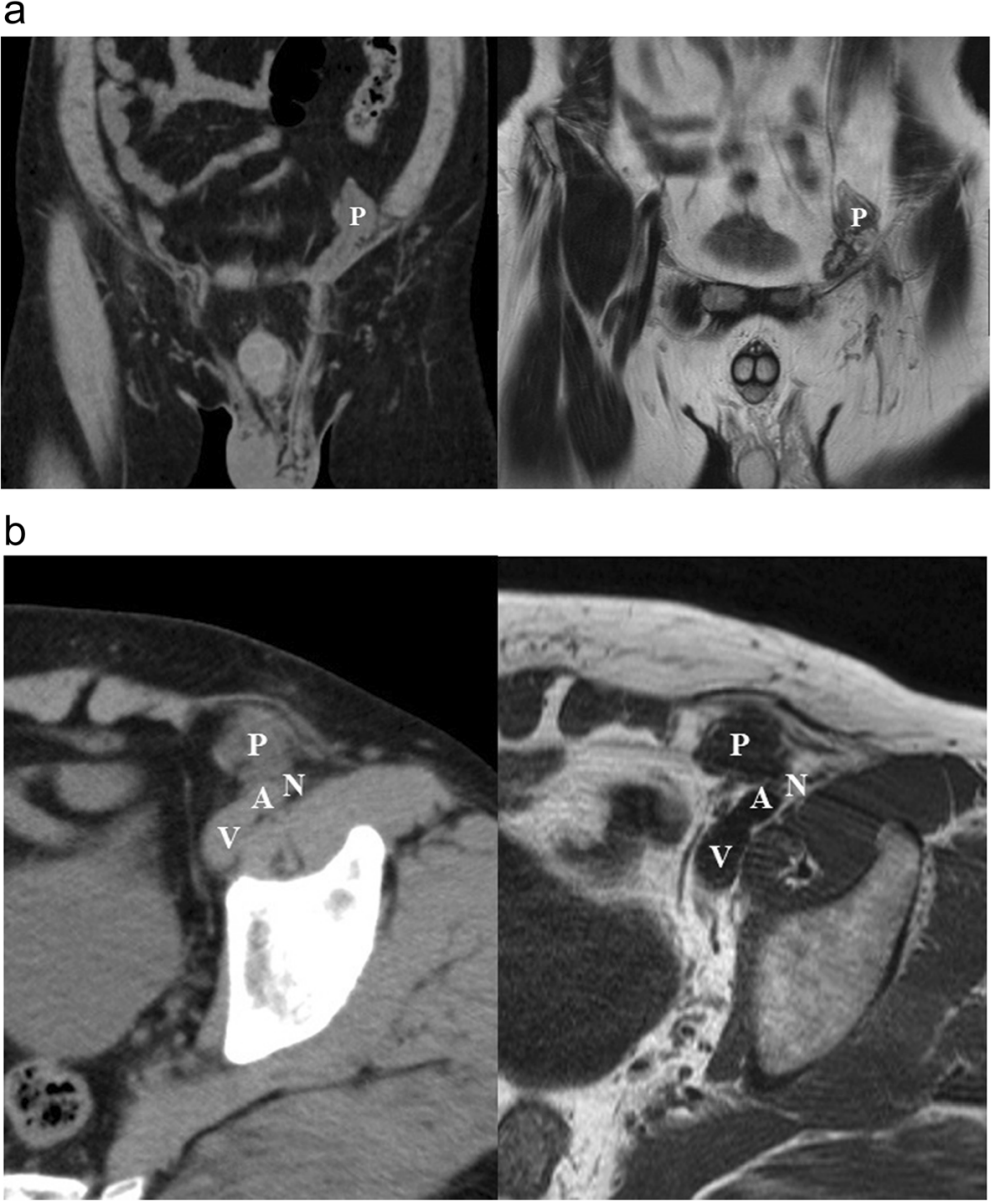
CT and MRI images. a Coronal computed tomography (CT) and magnetic resonance imaging (MRI) showing the plug bulging outward into the peritoneal cavity. P: plug. b Axial CT and MRI images showing the inward-projecting plug extremely close to the femoral nerve. A: external iliac artery (femoral artery); V: external iliac vein (femoral vein); N: femoral nerve; P: plug
